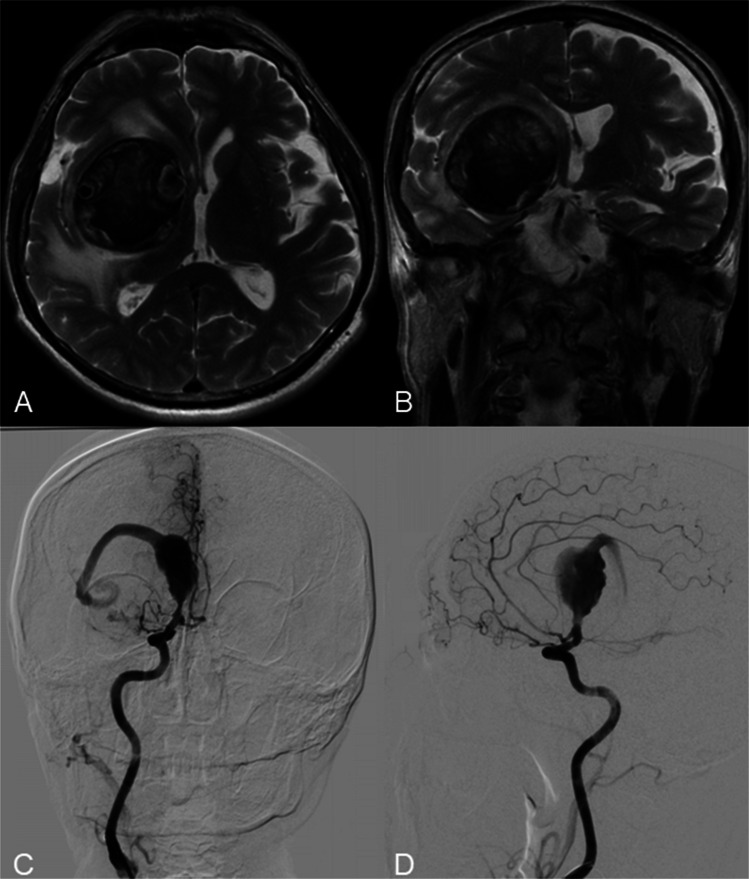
Axial (A) and frontal (B) T2-weighted brain MRI demonstrating the giant intracranial aneurysm with thrombosis in the lesion and surrounding edema. DSA from the right ICA (c, d) shows a giant partially thrombosed M1-2 segment fusiform aneurysm of the right middle cerebral artery
